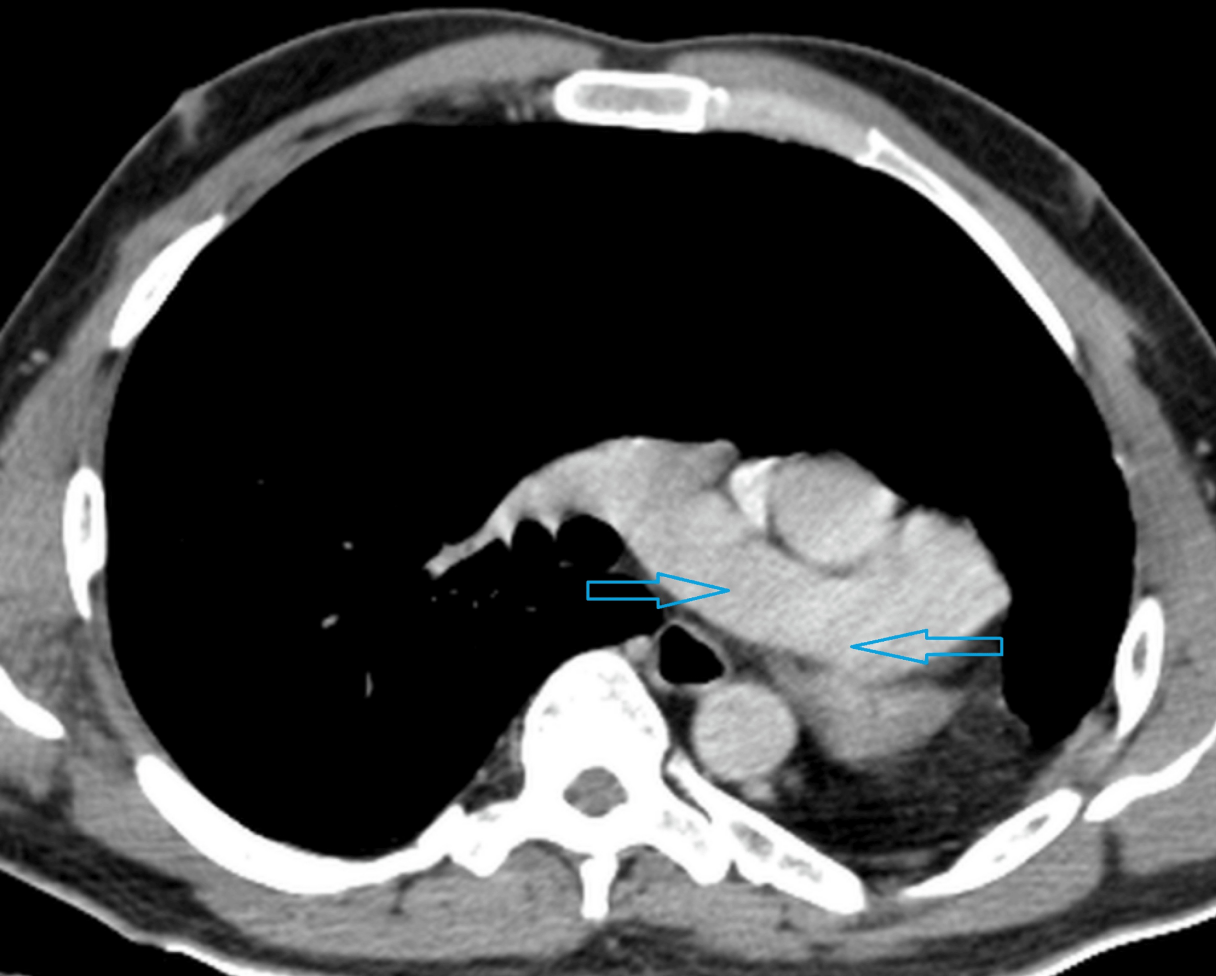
Contrast CT scan of the chest in the mediastinal window shows absence of the left pulmonary artery and normal appearing right pulmonary artery CT: computed tomography
